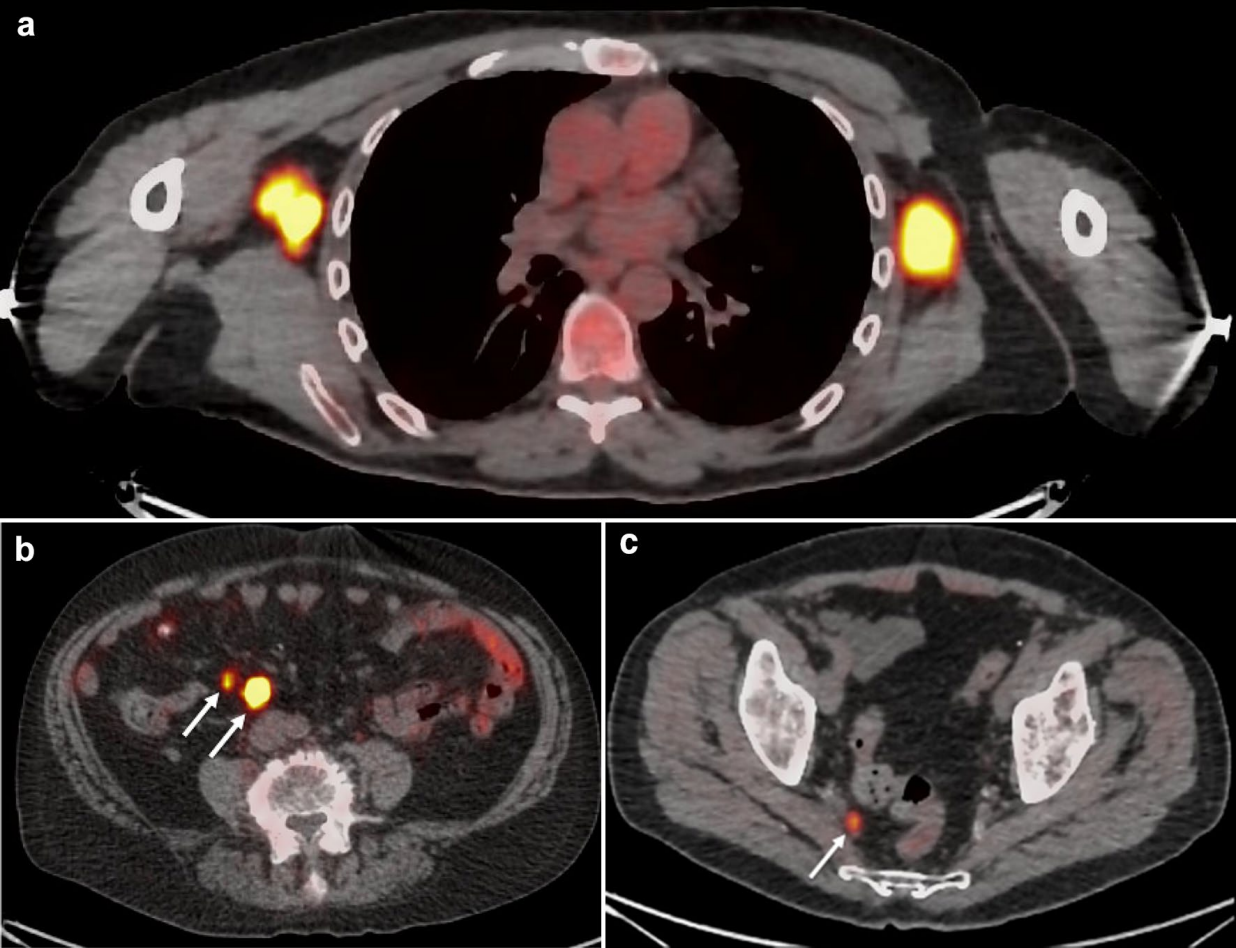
Axial fused PET-CT images of abnormal lymph nodes. FDG PET-CT (a) of bilateral axillary lymph node metastases from melanoma. DOTATATE PET-CT (b) of mesenteric nodal metastases from neuroendocrine tumor. Fluciclovine PET-CT (c) of a distal right internal iliac pelvic nodal metastasis from prostate cancer. Regardless of the radiotracer, the abnormal nodes appear as “hot spots.” For (b) and (c), the nodes are not large enough to be considered abnormal by CT alone
